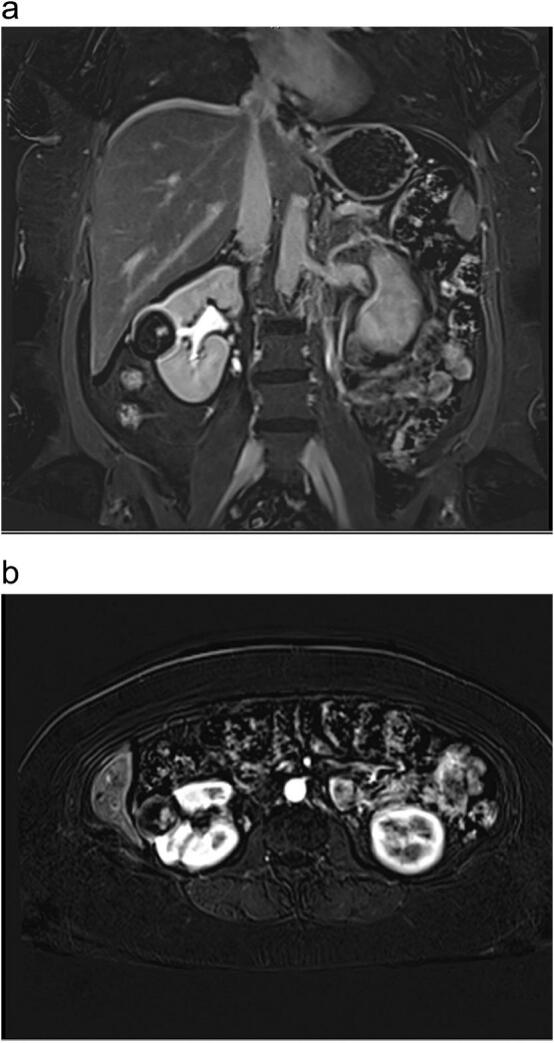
(a) and (b): MRI abdomen and pelvis showed interpolar region cortical exophytic 3.2 × 3.6 × 3.7 cm mass demonstrating low signal intensity on T2, with internal irregular nodule of high signal intensity on T1. There is reversal of signal intensity.
